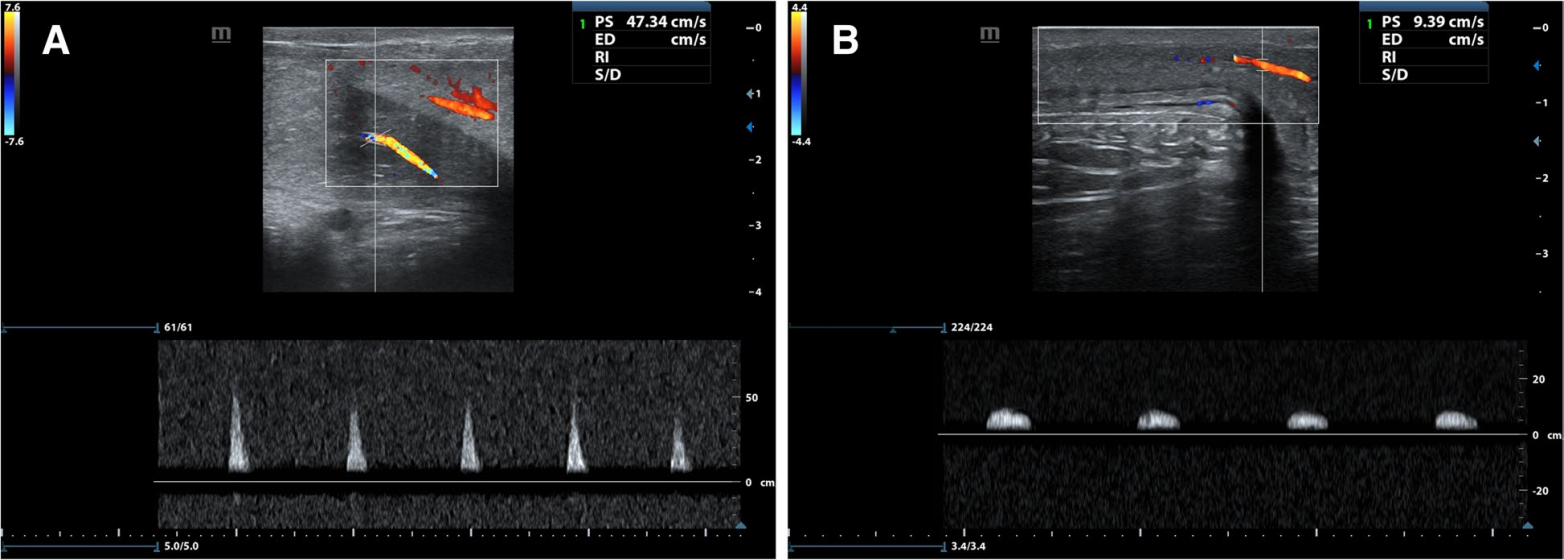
Longitudinal images acquired during penile Colour Doppler ultrasonography in two participants. Normal waveform with peak systolic velocity > 35 cm/s during the tumescent phase (A) in a participant without arterial insufficiency compared to a participant with peak systolic velocity < 25 cm/s (B)
